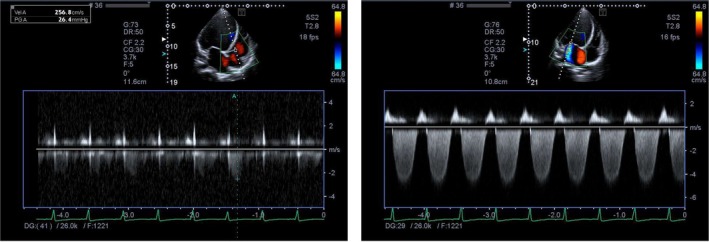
Pulsed‐wave Doppler and color and continuous‐wave Doppler showing regurgitant flow across the atrioventricular valves, consistent with moderate‐to‐severe functional mitral and tricuspid regurgitation secondary to ventricular dilation.
